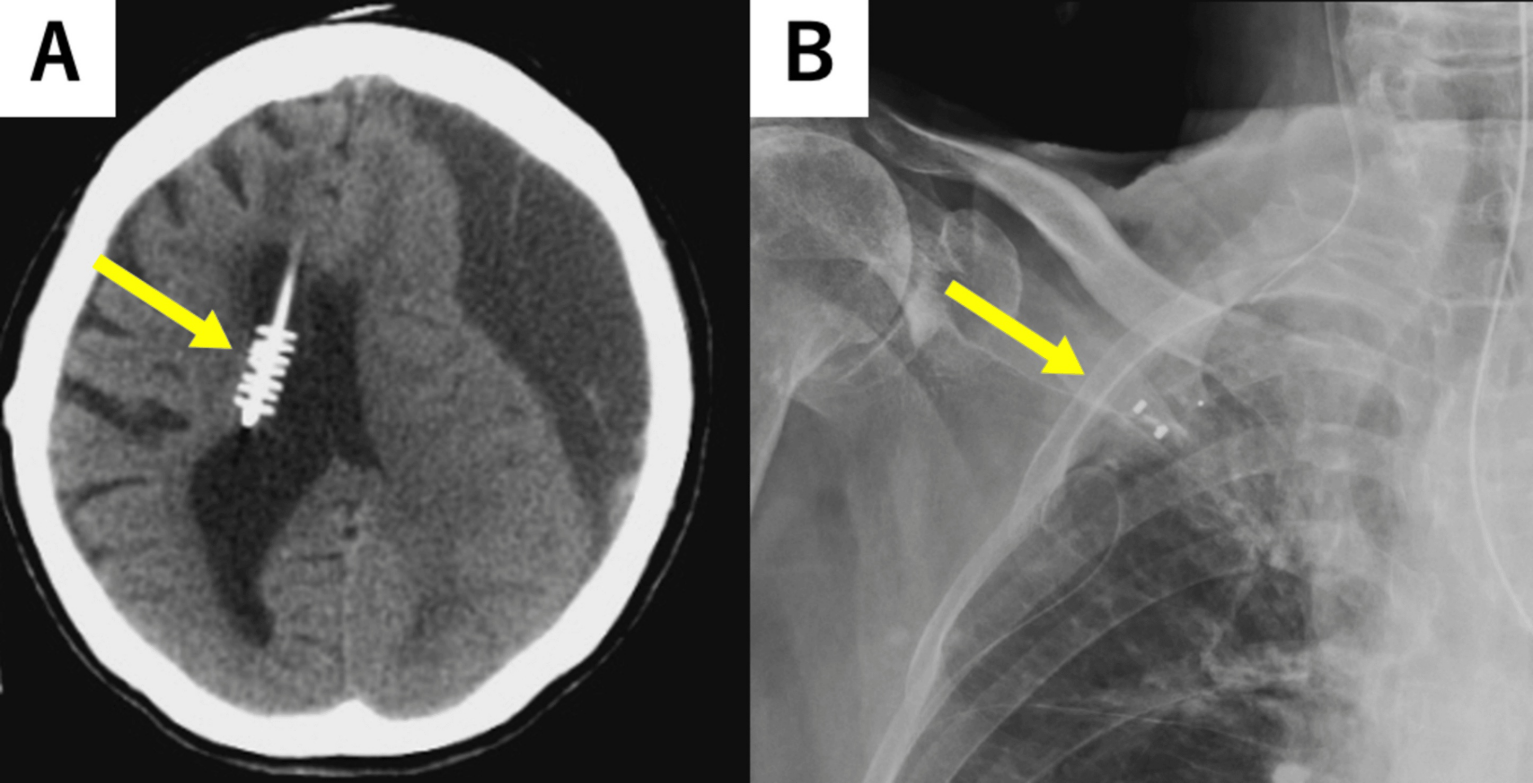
A: CT scan on admission; B: Plain chest radiograph after adding a valve A: A shunt was placed from the right anterior horn, and the tip of the tube had a flange shape. B: A programmable valve with a virtual off-function was added to the right anterior chest.
